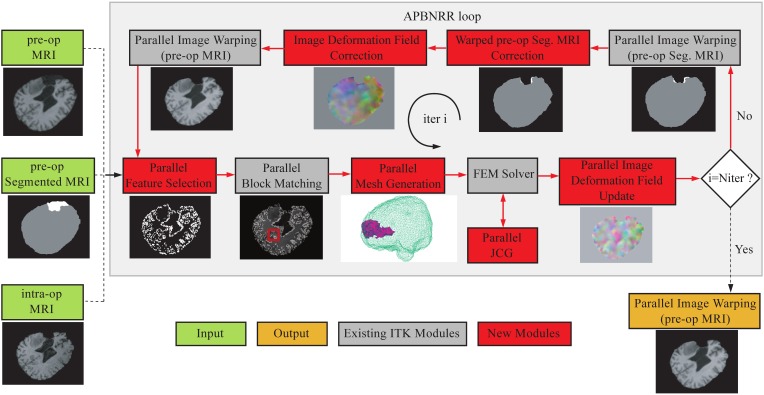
The APBNRR framework. The red boxes represent the new contributions and the gray boxes the existing ITK modules. The red arrows show the execution order of the different modules. The APBNRR loop breaks when the desired number of iterations has reached. The output image (orange box) is the warped pre-op MRI when *i* = *N*_iter_.
